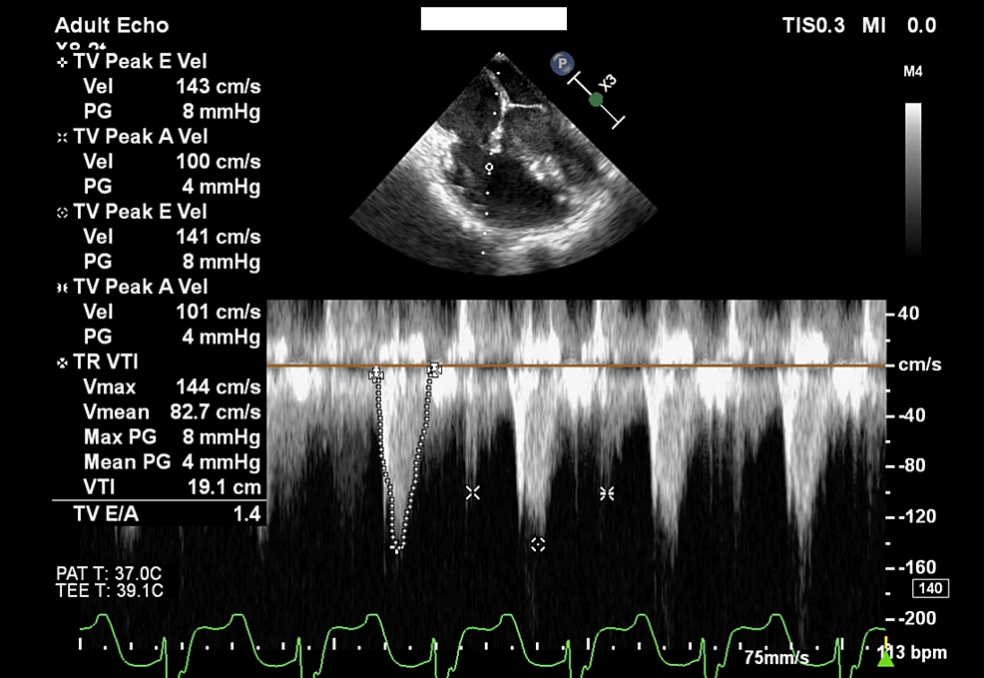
Four-chamber view from intraoperative TEE after cone reconstruction of the TV. TV mean PG was 4 mmHg and max PG was 8 mmHg. TEE: transesophageal echocardiography; TV: tricuspid valve; PG: pressure gradient
